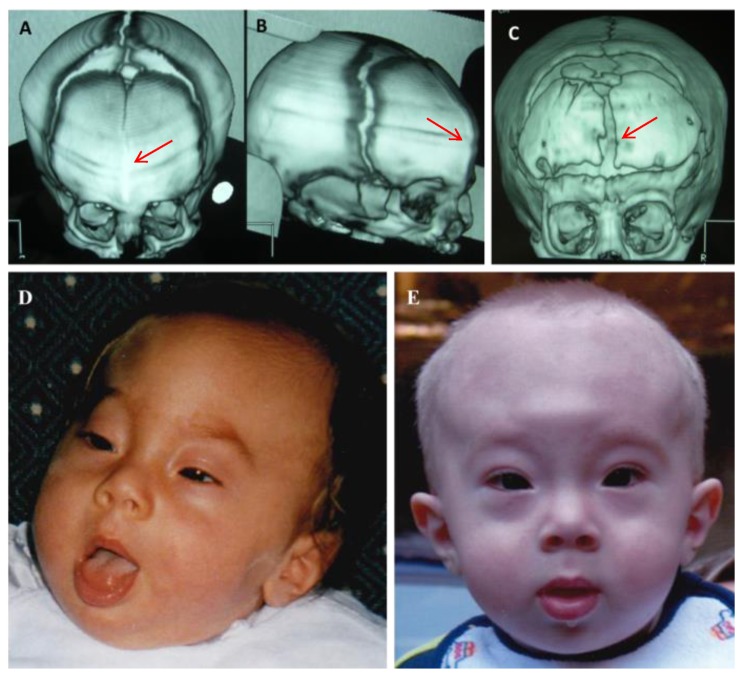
Computed tomography (CT) scan of the cranium with helical and 3D reconstruction. (A,B) One month old: skull morphology appears triangular; the metopic suture is already calcified in its middle-lower portion (red arrows), and gives the skull a trigonocephalic aspect; (C) Eleven months old: results of bilateral frontal bone craniotomy with removal of the median hyperostotic ridge (red arrow); (D) Patient one month old, showing a high forehead, a prominent metopic suture, bitemporal narrowing, up-slanting palpebral fissures, and anteverted nares; (E) Patient eight months old, after the craniotomy, has a wider bitemporal space and less pronounced up-slanting palpebral fissures.
